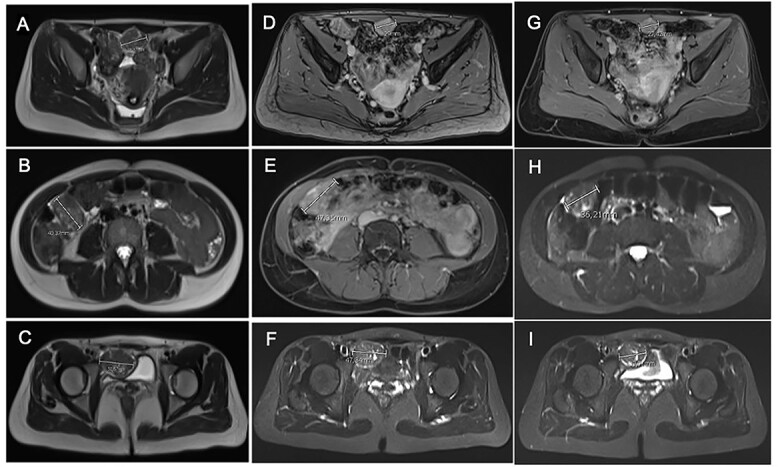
Comparative magnetic resonance imaging (MRI). (A–C) MRI after 3 months of acetate ulipristal. (A) Tumor in contact with infra-umbilical linea alba; (B) lesion located in right hypochondria; (C) tumor situated in right iliac fossa near bladder. (D–F) MRI after 3 months of AI and GnRH agonist. (G–I) preoperative MRI.
